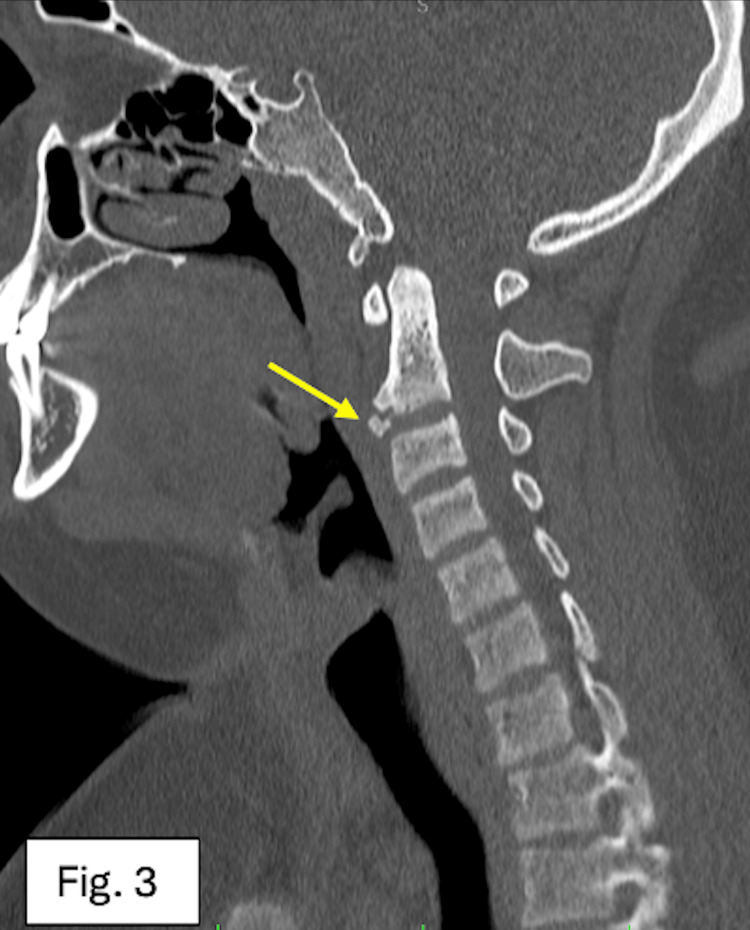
Sagittal CT cervical spine in bone window showing a small well-corticated bony fragment (arrow) at the anteroinferior aspect of the C2 vertebra.
